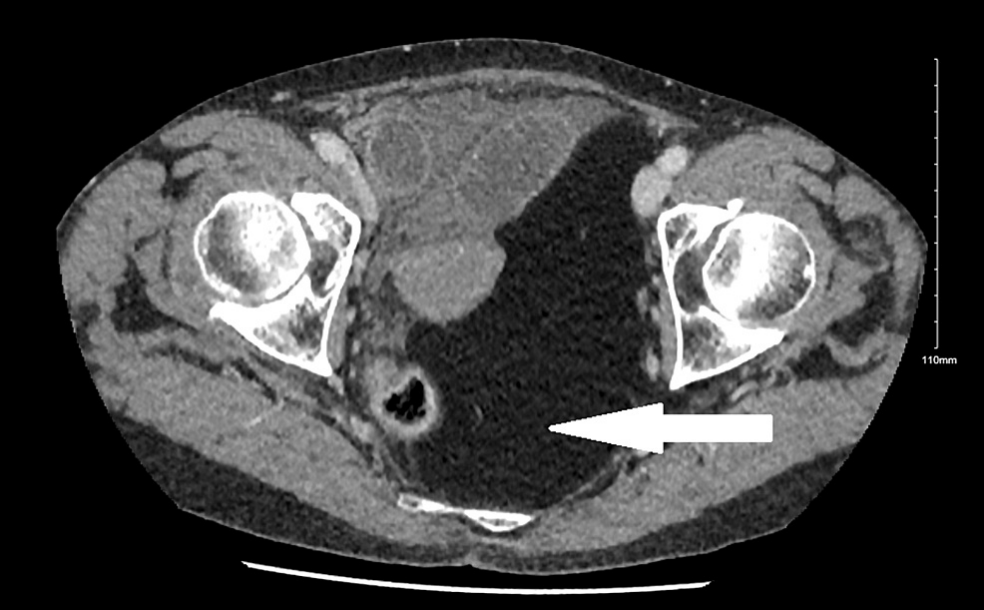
Axial view left-posterior intra-pelvic lipoma on computed tomography abdomen and pelvis.
